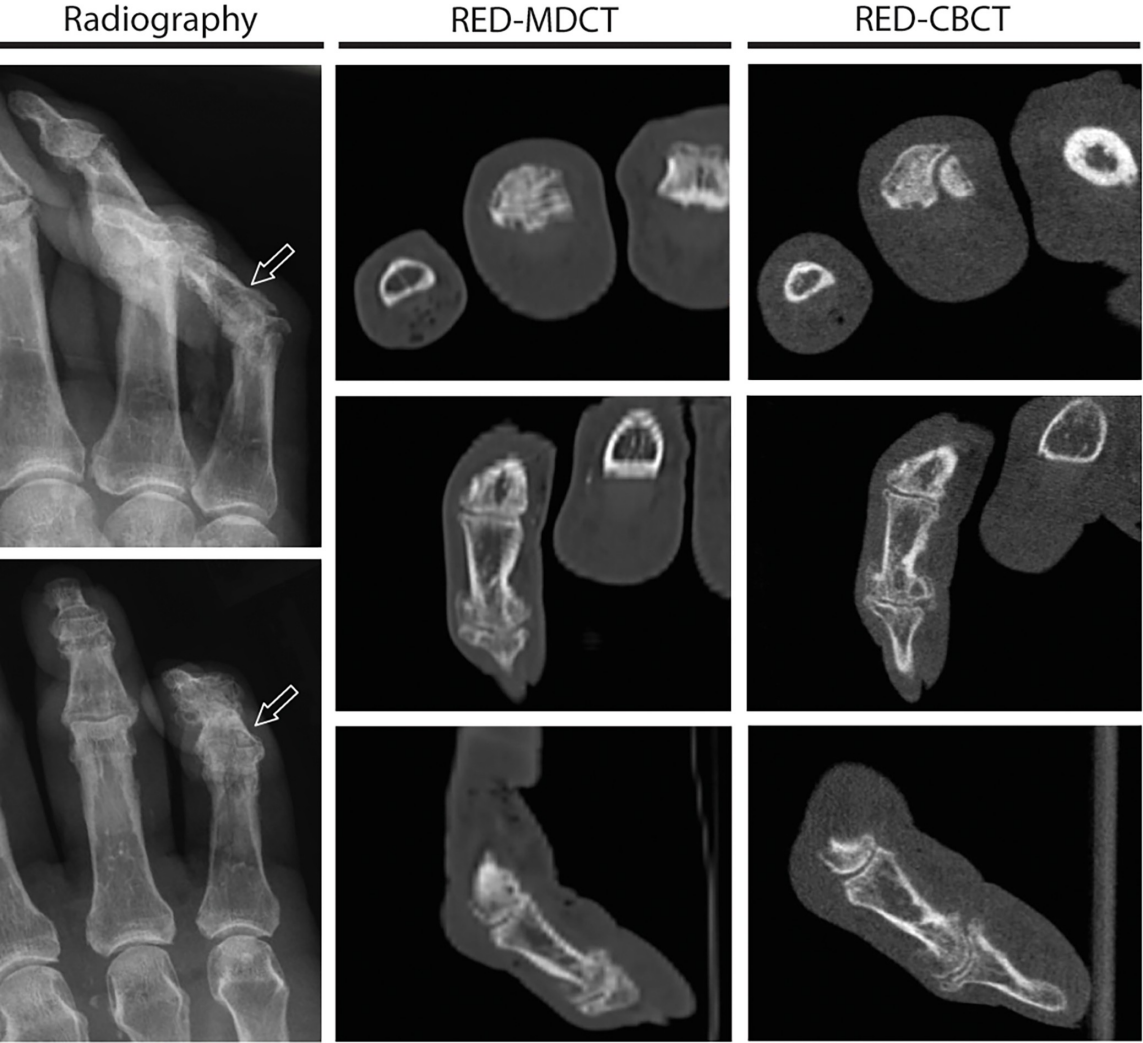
Raters suspected a fracture in radiography in Phalanx media of Digitus V that didn´t exist and therefore didn´t show up in the RED-CT images. Imaging examples with axial, coronal and sagittal reconstructions (top down) for radiography (first column, see arrow pointing towards the suspected fracture), RED-MDCT (second column) and RED-CBCT (third column).
